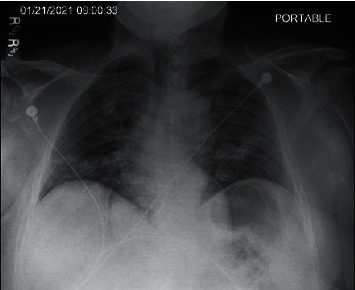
Chest X-ray: patchy bilateral infiltrates.
